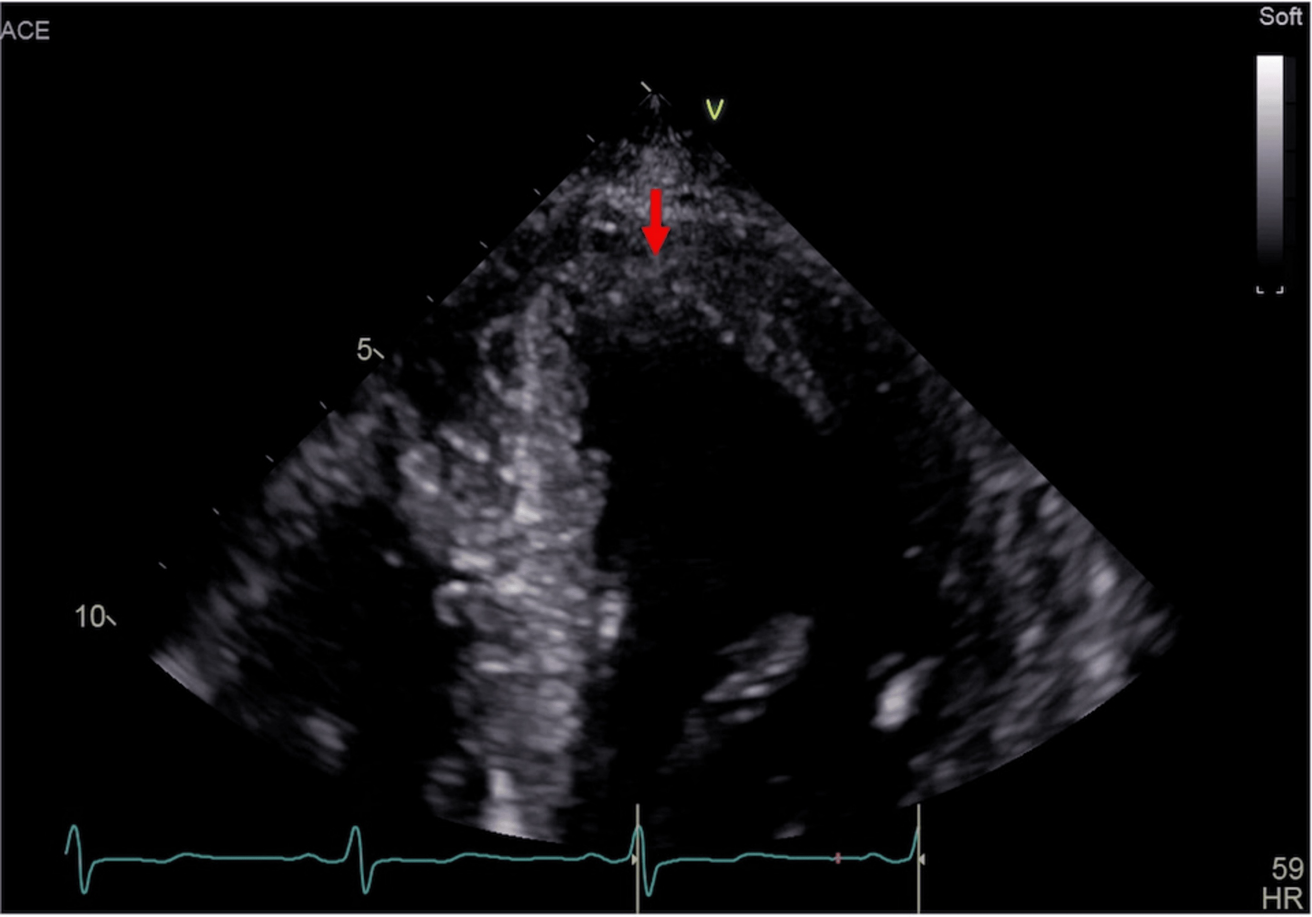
Disappearance of left ventricular thrombus (parasternal short axis view apical level) Transthoracic echocardiography performed five months after the surgery showing the disappearance of the thrombus (red arrow)
